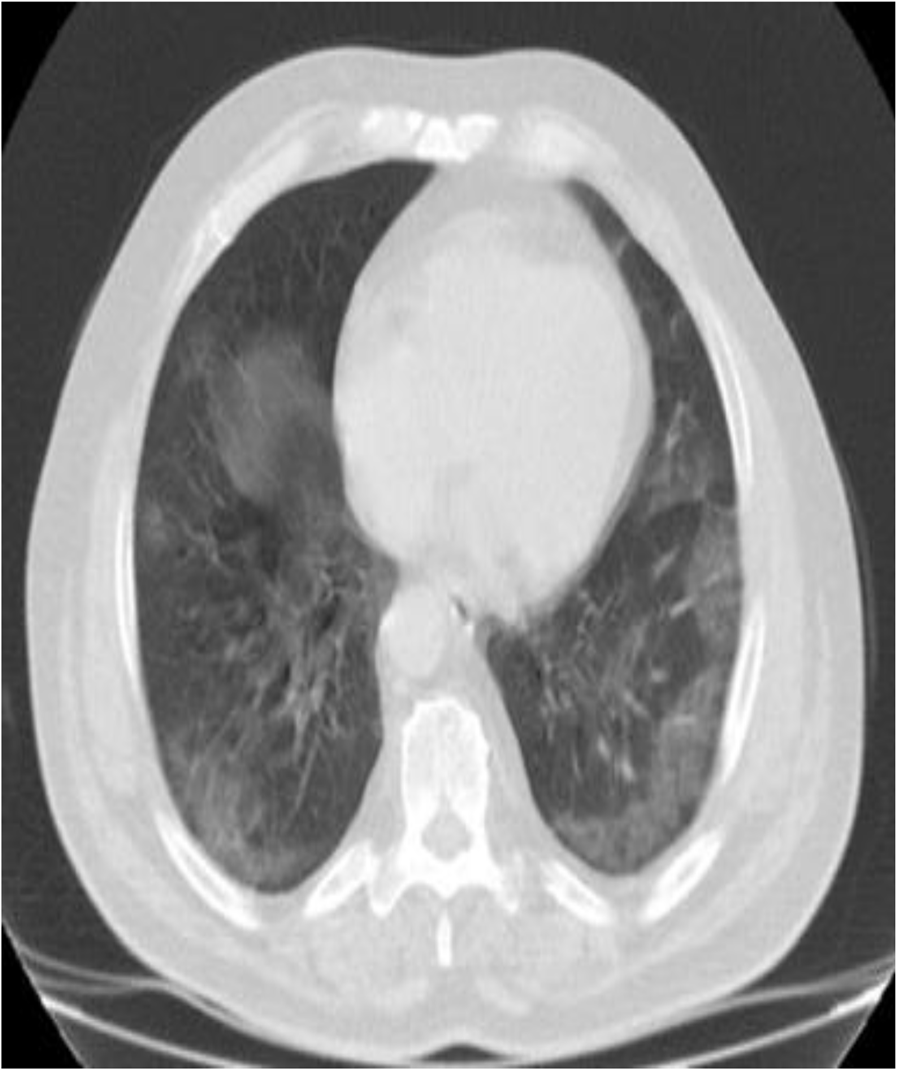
Chest CT findings on admission
